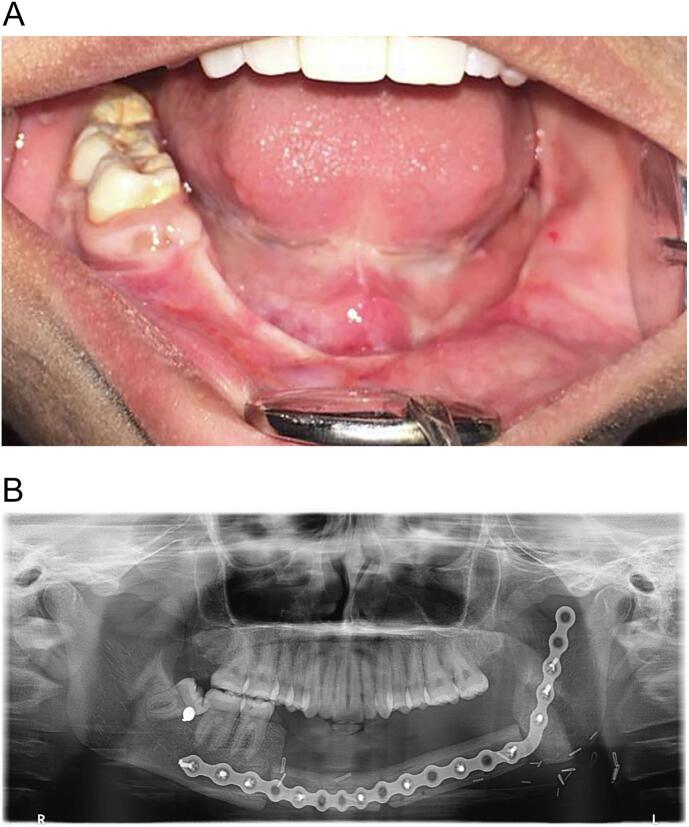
The patient's clinical presentation. A. The intraoral view presents reconstructed mandibular resected bone associated with an obliterated sulcus, complete maxillary teeth, and 46, 47, and 48 teeth. B. The panoramic radiograph reveals a free fibula flap, which has replaced the segmental mandibulectomy, along with a reconstructive plate and a horizontally tilted 48 tooth.
